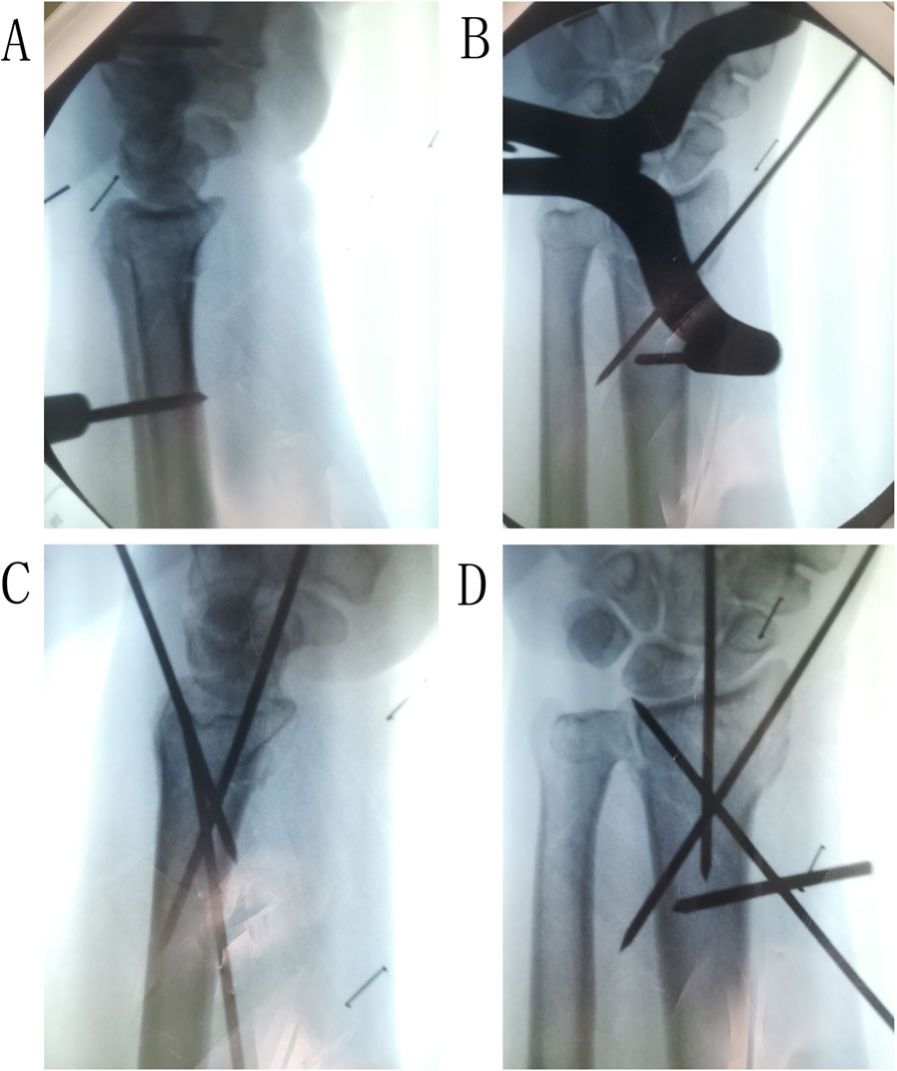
After a sound anatomic reduction was achieved (A, B), three 1.8 Steinmann pins (Styloid process pin, Lister tubercle pin, Sigmoid notch pin) were inserted in sequence and were expected to achieve a cross-distribution in the frontal and lateral X-ray films (C, D). The radial height (11.33mm), radial inclination (21.44°), volar tilt (10.32°), the radial shift (+0.26mm), and ulnar variance (−1.22mm) were also confirmed improved (C, D)
